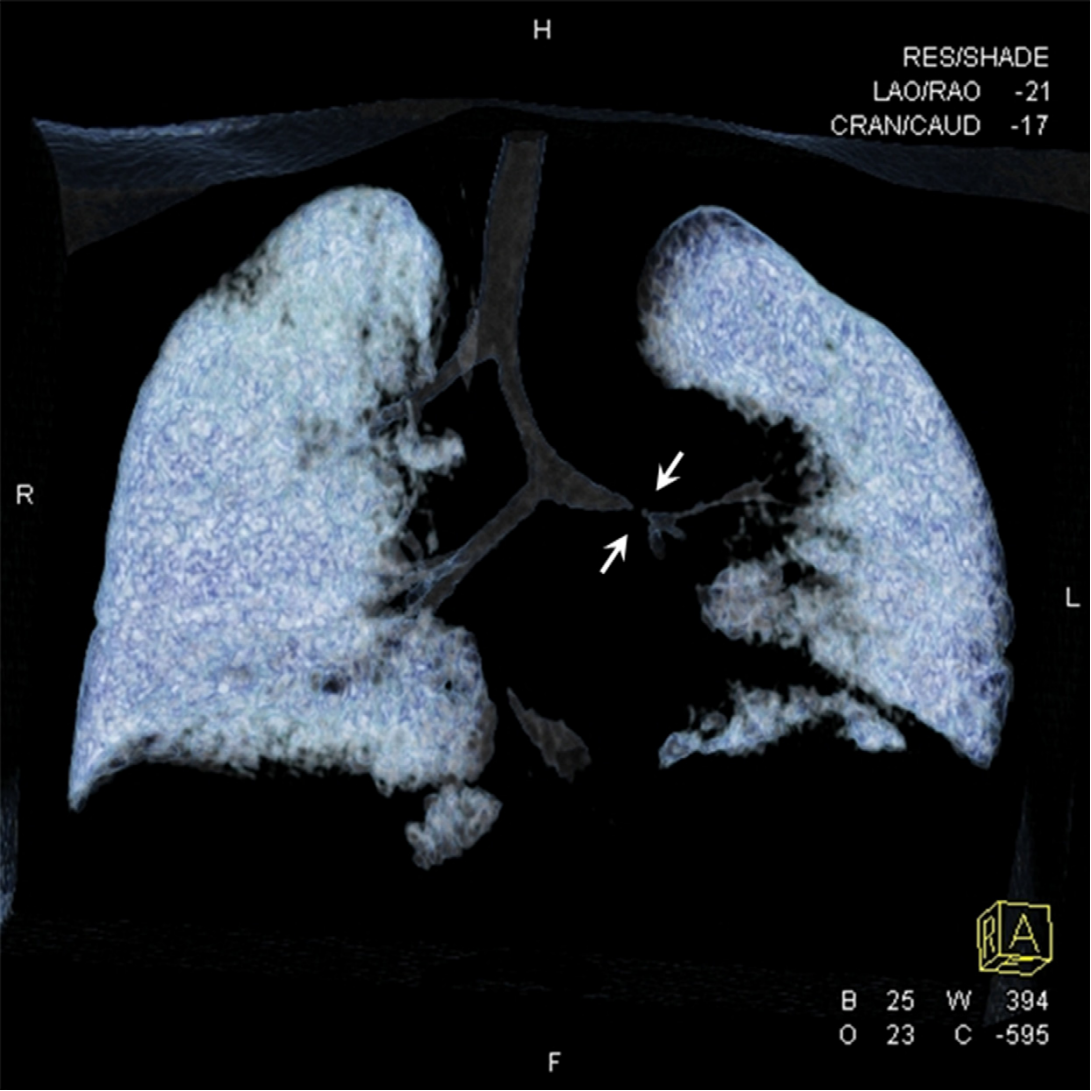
Computed tomography revealed peculiar bronchial anatomy and severe left main bronchial stenosis.
